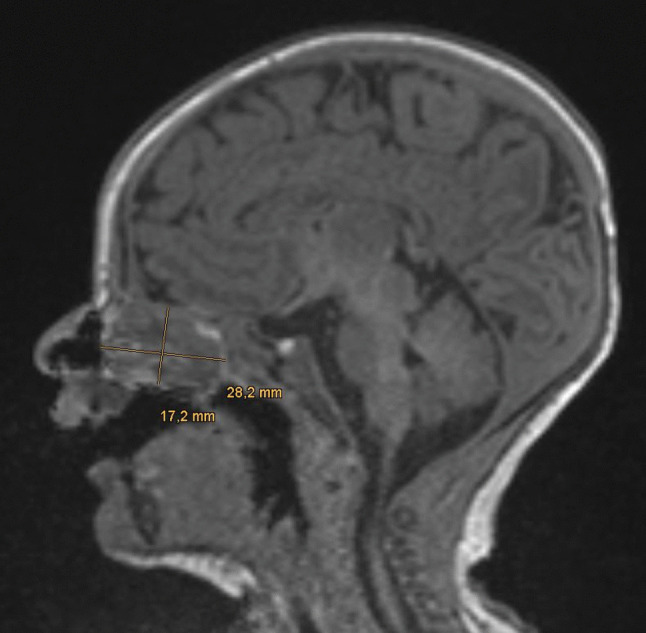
Expansion of Kaposiform Haemangioendothelioma in T1 weighted MRI scan in sagittal plane
